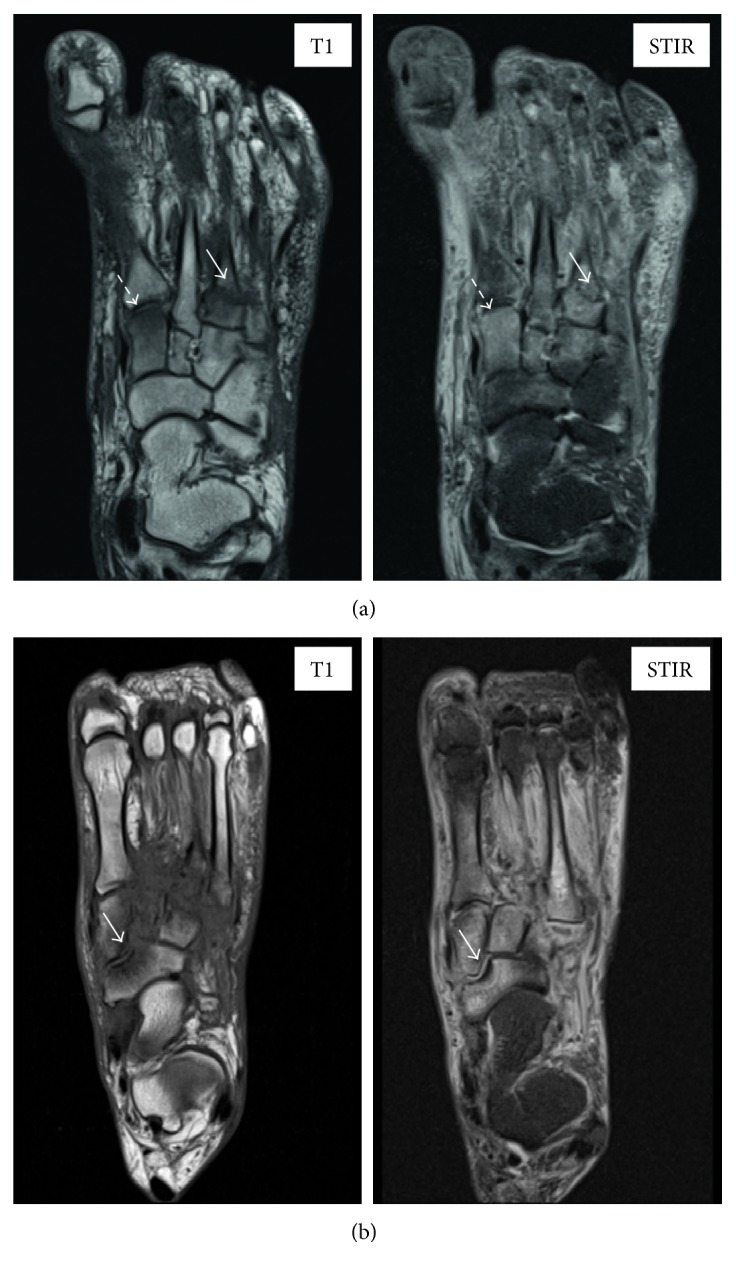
Noncontrast large field-of-view MR scans in active Charcot osteoarthropathy. Representative examples of bone marrow oedema at the medial cuneiform (BMO score = 2; white dashed arrow) and fracture at the base of the 3rd metatarsal (fracture score = 1; white arrow) noted on axial T1 and STIR MR images (a). Example of collapse of the navicular bone (fracture score = 2, white arrow) noted on axial T1 and STIR MR images (b).
